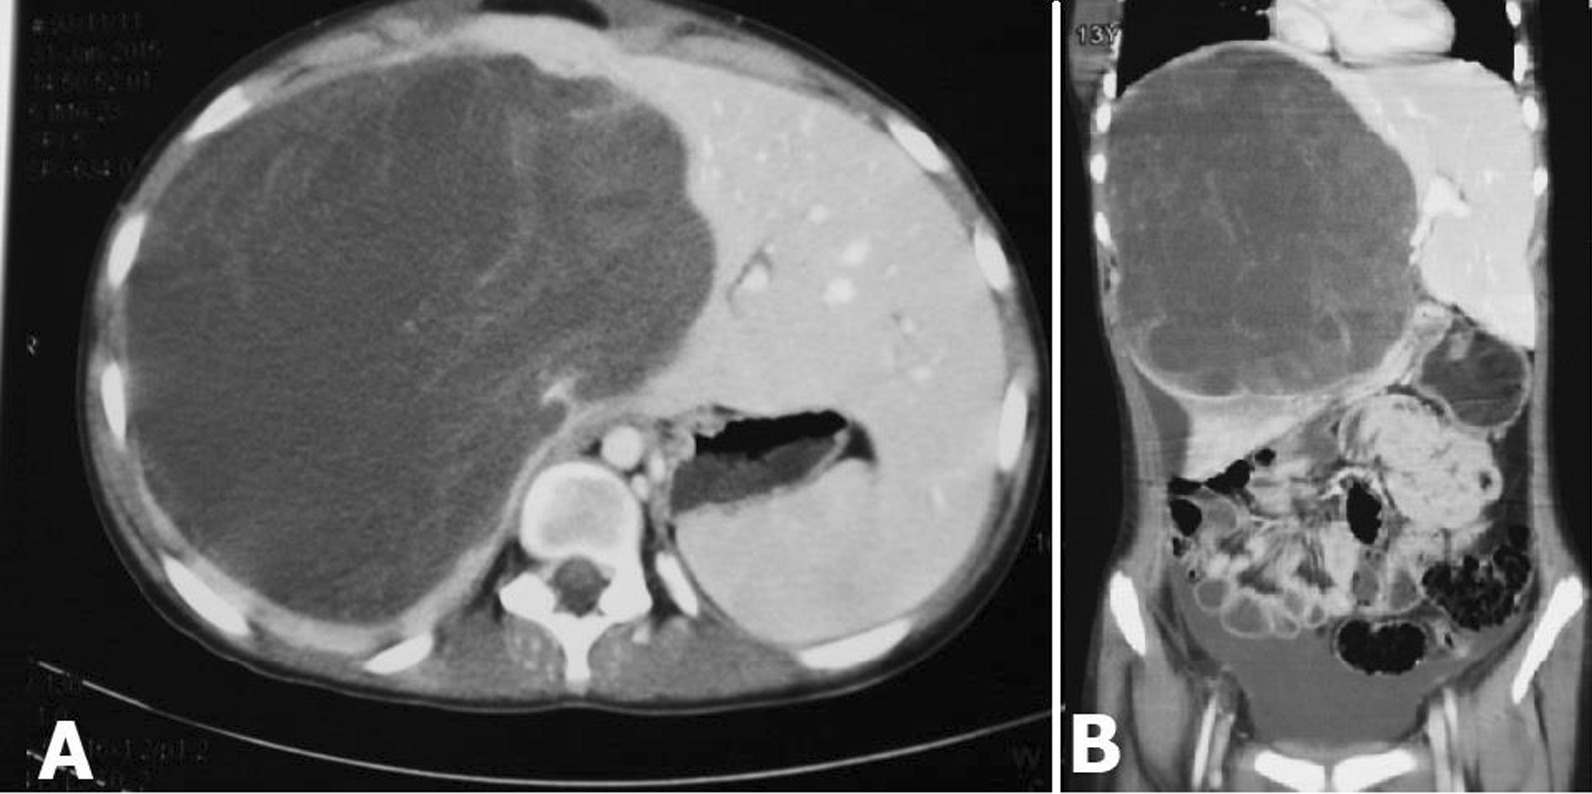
Computed tomography images of a hypodense lesion in the right lobe of the liver, in favor of a huge undifferentiated embryonal sarcoma of the liver
